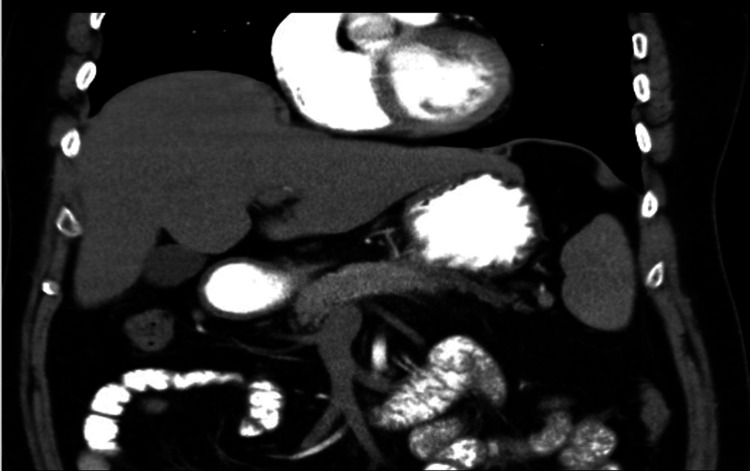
CT abdomen after treatment (coronal view) The CT showed complete resolution of pancreatic lesions.
